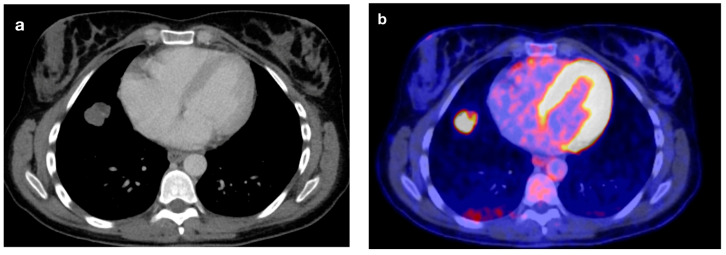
(a) A 31-year-old female patient with malignant melanoma and an initial suspicion of pulmonary hamartoma due to presumably macroscopically visible fat without a DECT follow-up. (b) The subsequent PET-CT a few weeks later shows an intensive FDG image. The lung lesion ultimately turned out to be the metastasis of a malignant melanoma.
